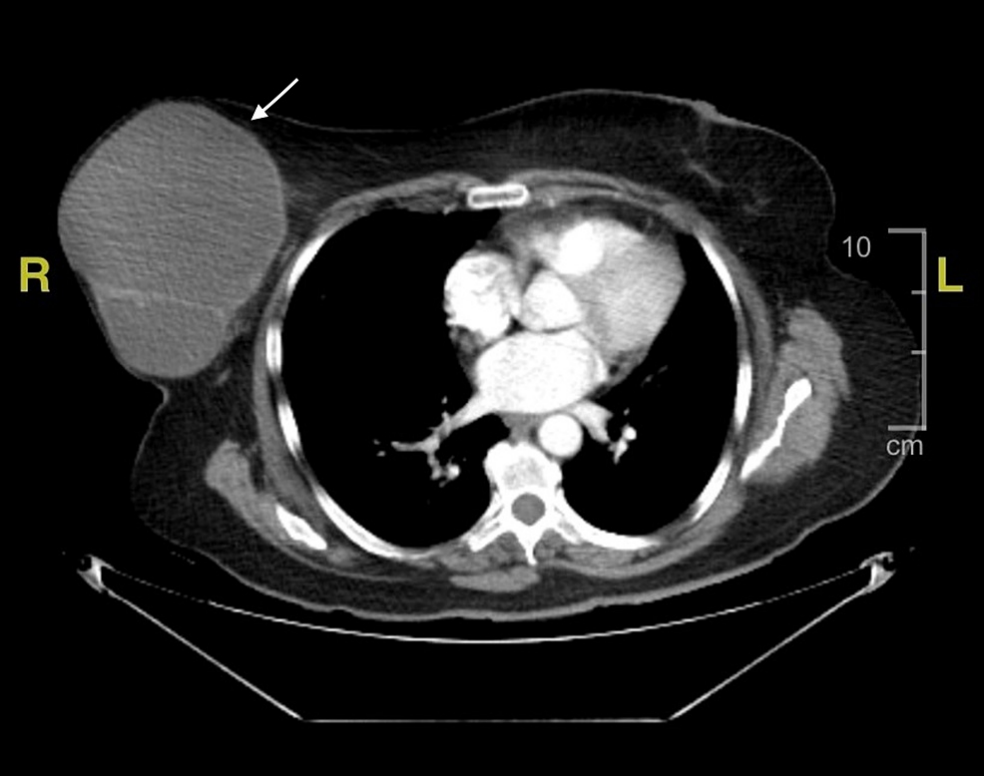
Axial enhanced CT imaging of the thorax. The image demonstrates a right-sided solid mass lesion (white arrow) measuring 15.2cmX11.8cmX 15.2cm. The mass is septated, predominantly cystic, and contains solid, irregularly-shaped polypoid components.
